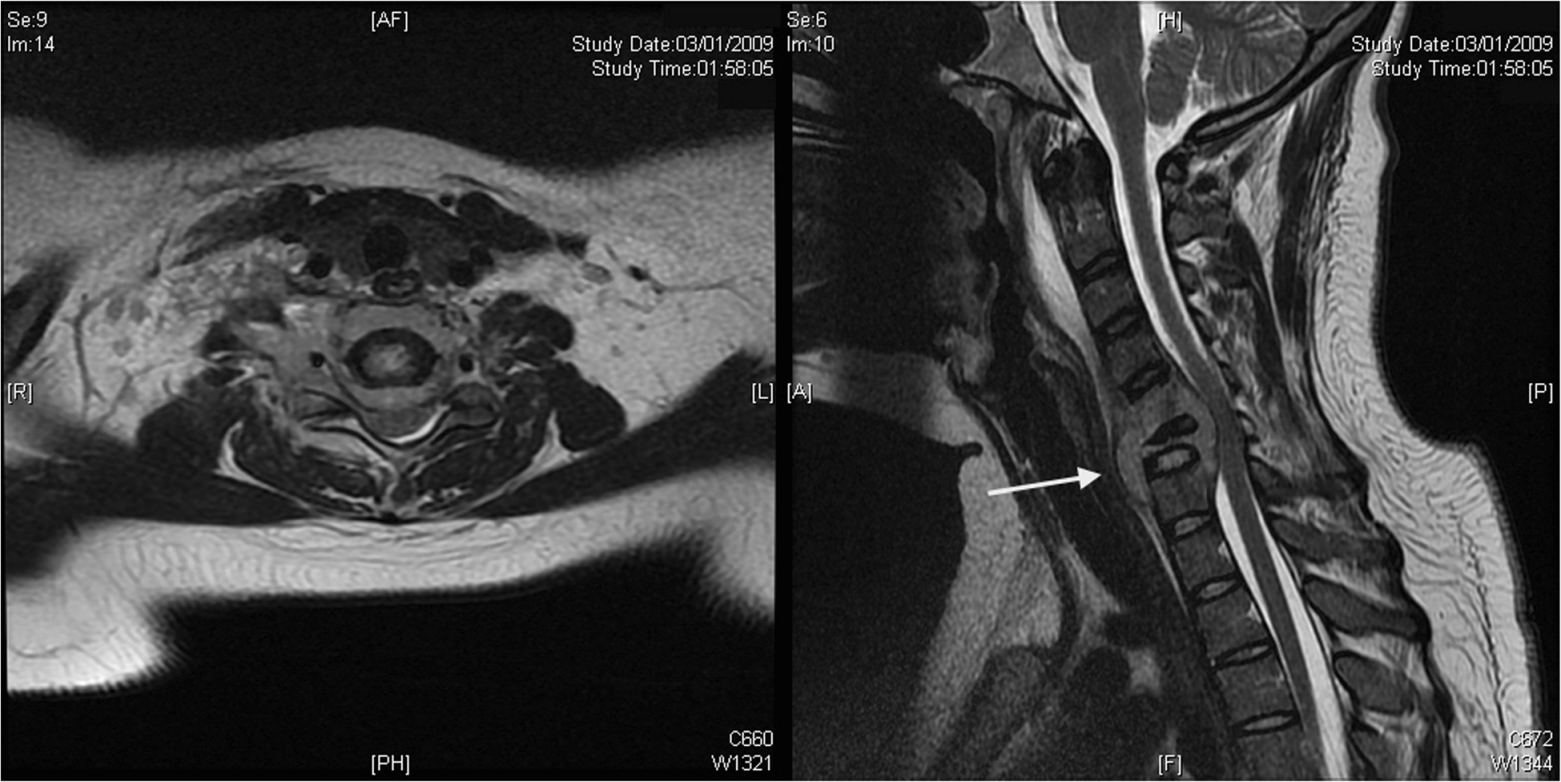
Preoperative sagittal (*right*) and axial at the C6 level (*left*) T2-weighted fast spin-echo magnetic resonance imaging views. The views show C6 osteomyelitis with significant vertebral body destruction (arrow), vertebral abscess at C6 level with posterior subligamentous extension into the spinal canal including mild posterior displacement and compression of cervical spinal cord
